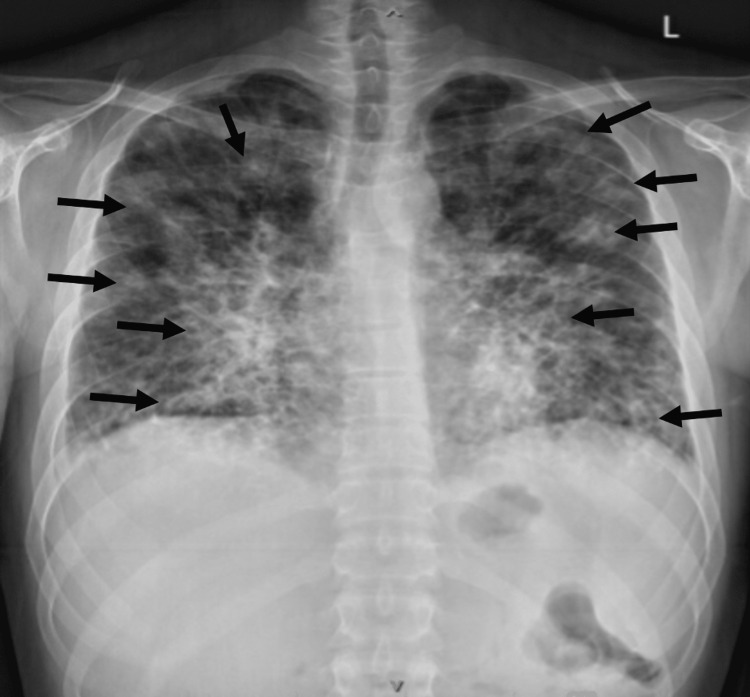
Chest X-ray with multifocal bilateral airspace opacities.
